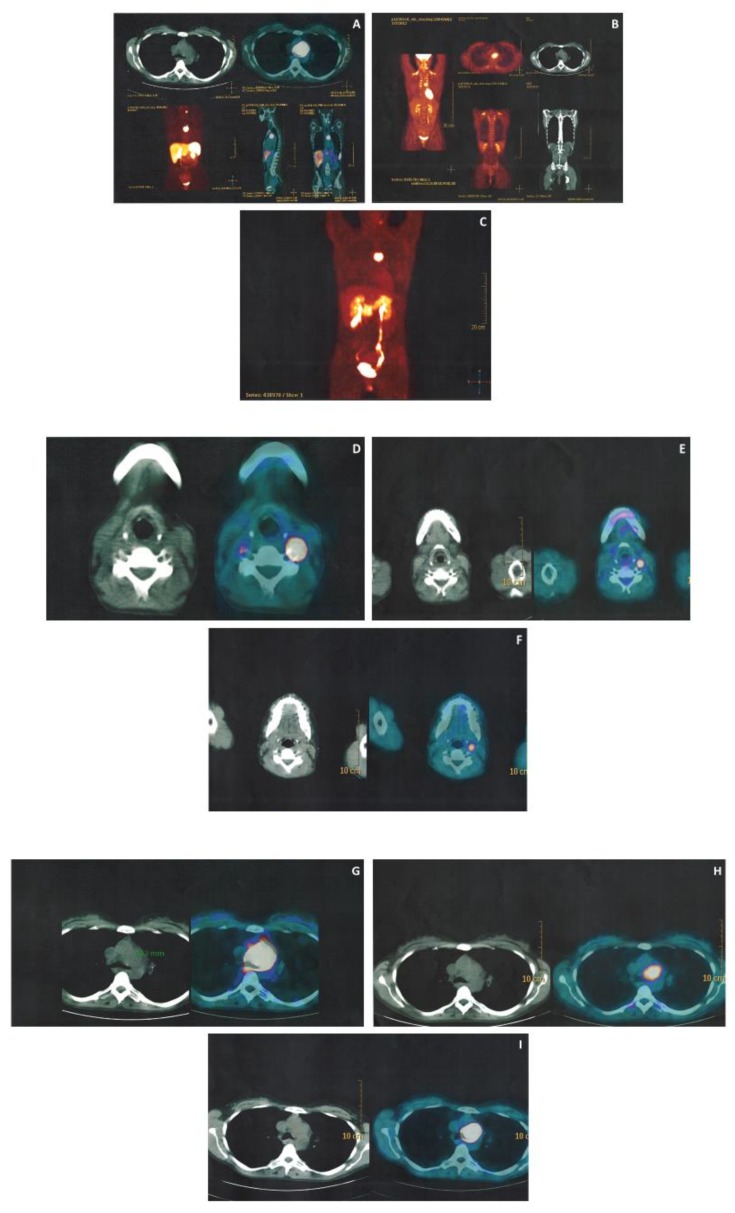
PET-CT comparison: (A,D,G): 68GaDOTATOC; (B,E,H): 18F-FDG; (C,F,I): 18F-DOPA. Abbreviations: PET = positron emission tomography; CT = computed tomography; DOTATOC = 1,4,7,10-tetraazacyclododecane-1,4,7,10-tetraacetic acid tyr3- Octreotide; FDG = fluoro-2-deoxy-D-glucose; DOPA = 3,4-dihydroxyphenylalanine.
